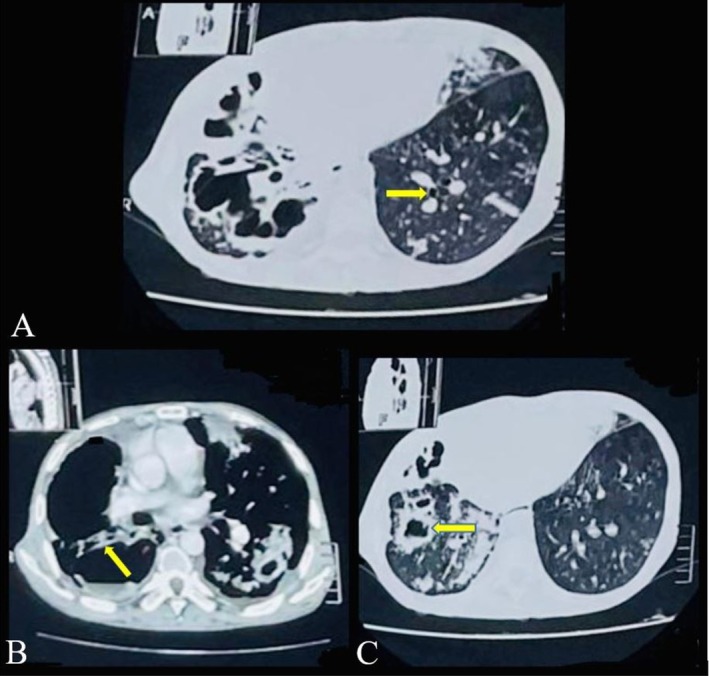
Axial chest CT images demonstrating extensive bilateral structural lung disease consistent with post‐tuberculosis sequelae*. (A) Cystic bronchiectasis with clustered dilated airways showing a signet ring appearance. (B) Tram‐track appearance of bronchiectasis with thickened dilated bronchi. (C) Large bullae, more prominent in the right lung.
